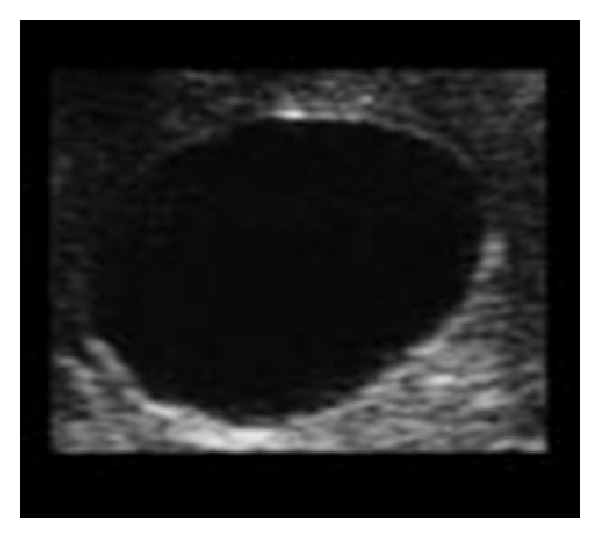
Ultrasound image of axillary cyst.
